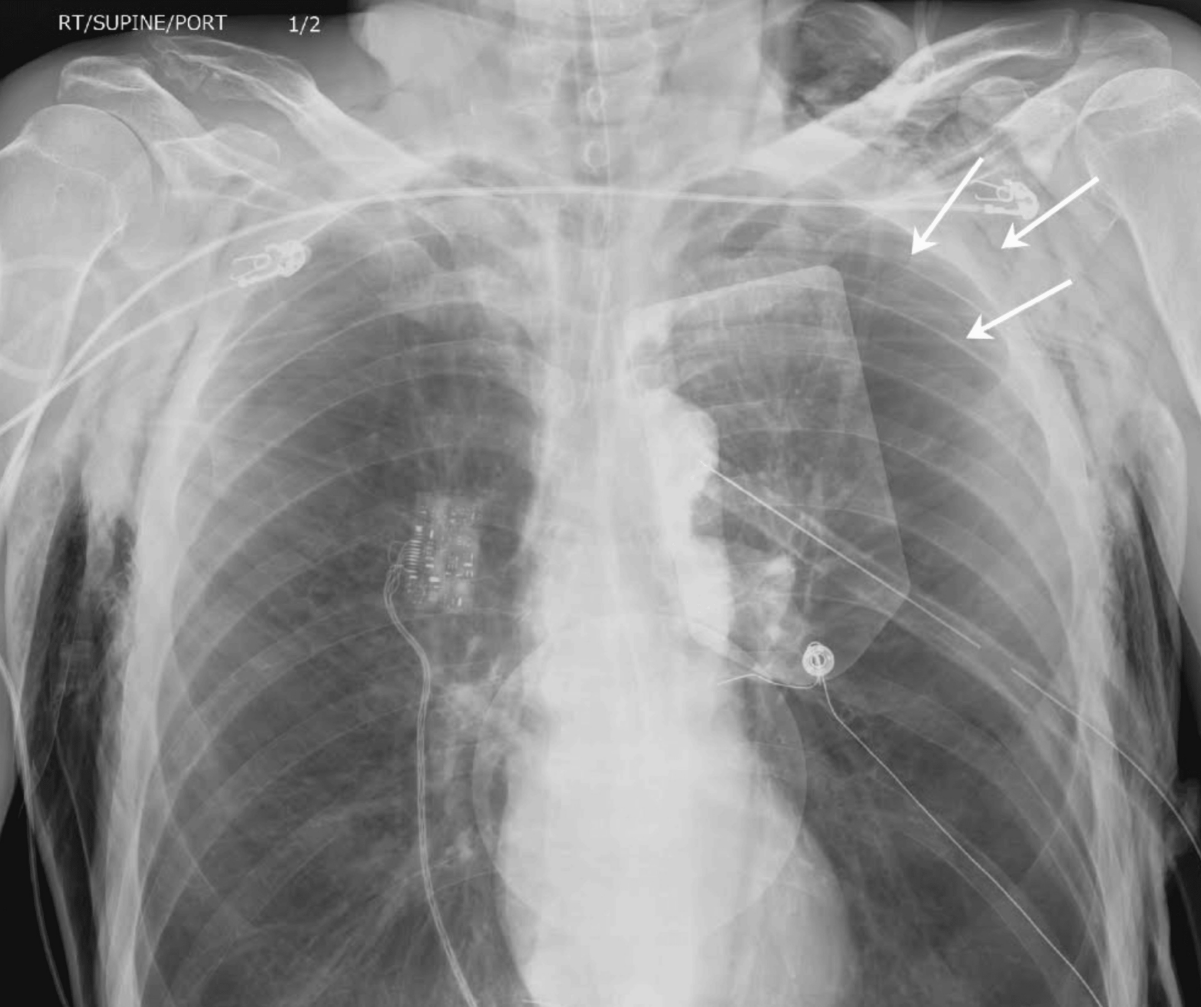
The chest X-ray demonstrates left pneumothorax (arrows) and lucency projecting over the left scapula suggestive of a non-displaced fracture. A chest tube and defibrillation pad can be seen on the left. Telemetry lead is seen on the right.
